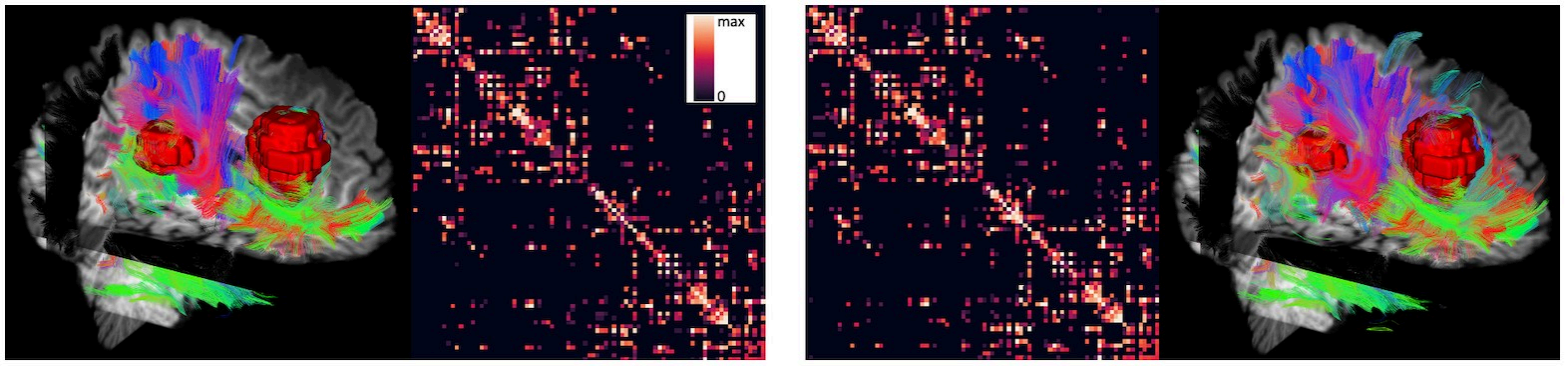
Tractography result and fiber-count connectivity matrix with (right) and without (left) a free water model for a brain tumor patient with a large edema. Here, gray matter interface seeding was used together with deterministic tractography. For better visualization, only every 4th streamline is plotted. The tumor core is visualized in red, and displayed together with three T1 slices in x,y, and z direction as well as streamlines generated from the diffusion MRI data.
